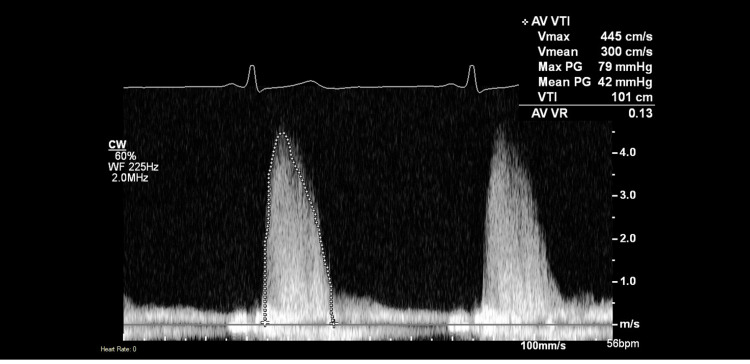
Preoperative TTE With Continuous Wave Doppler Demonstrating Severe Aortic Stenosis. The spectral Doppler waveform shows a characteristic dense, dagger-shaped envelope with markedly elevated transvalvular velocities. Hemodynamic parameters include peak aortic jet velocity (V_max_) of 445 cm/s, mean velocity (V_mean_) of 300 cm/s, peak pressure gradient of 79 mmHg, mean pressure gradient of 42 mmHg, and velocity time integral (VTI) of 101 cm. Minimal aortic regurgitation is present with a valve regurgitation (VR) ratio of 0.13. These findings meet diagnostic criteria for severe, high-gradient aortic stenosis (mean gradient >40 mmHg, peak velocity >4 m/s) and provide a clear indication for surgical aortic valve replacement in this young, symptomatic patient with bicuspid aortic valve disease. TTE: transthoracic echocardiography
